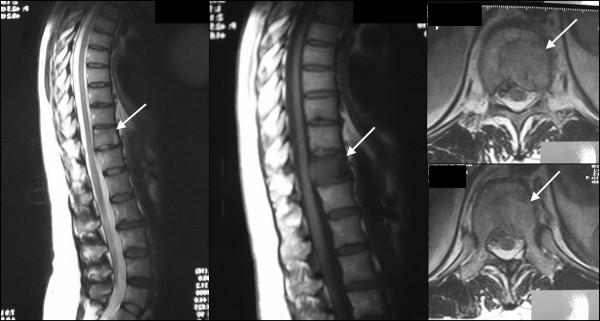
On MRI this expansive lesion with low signal in T1 WI and high signal in T2 WI conditioned left root compression and enhanced after gadolinium injection. These features suggest the diagnosis of a malignant lesion, most probably a metastasis. Arrow indicating the lesion.
